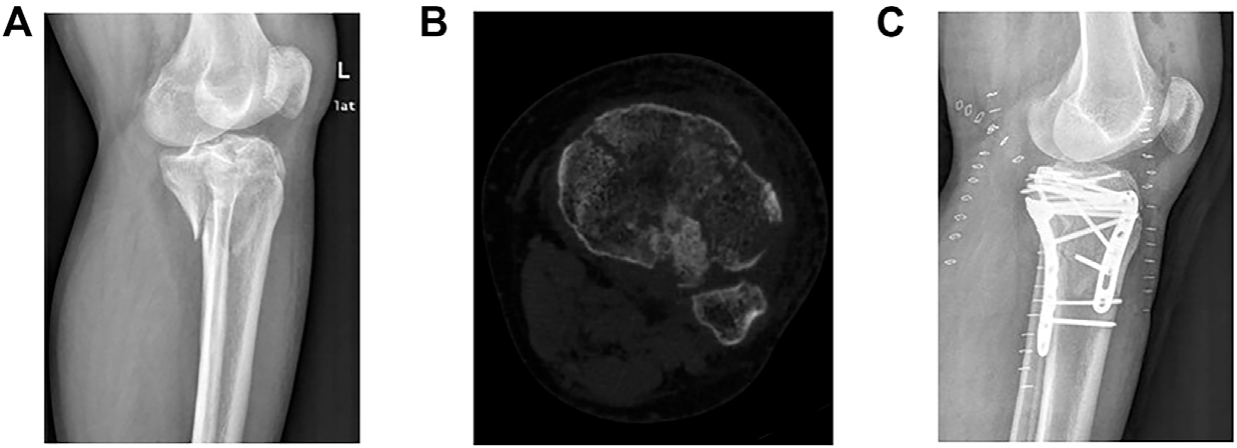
Imaging examinations of the affected limb before and after surgery. (A) Preoperative lateral X-ray of the affected limb, showing posterolateral fracture fragments. (B) Transverse CT of the affected limb, showing fractures of the lateral, medial, and posterior columns. (C) Postoperative lateral X-ray of the affected limb, showing good joint surface reduction and fracture fixation.
